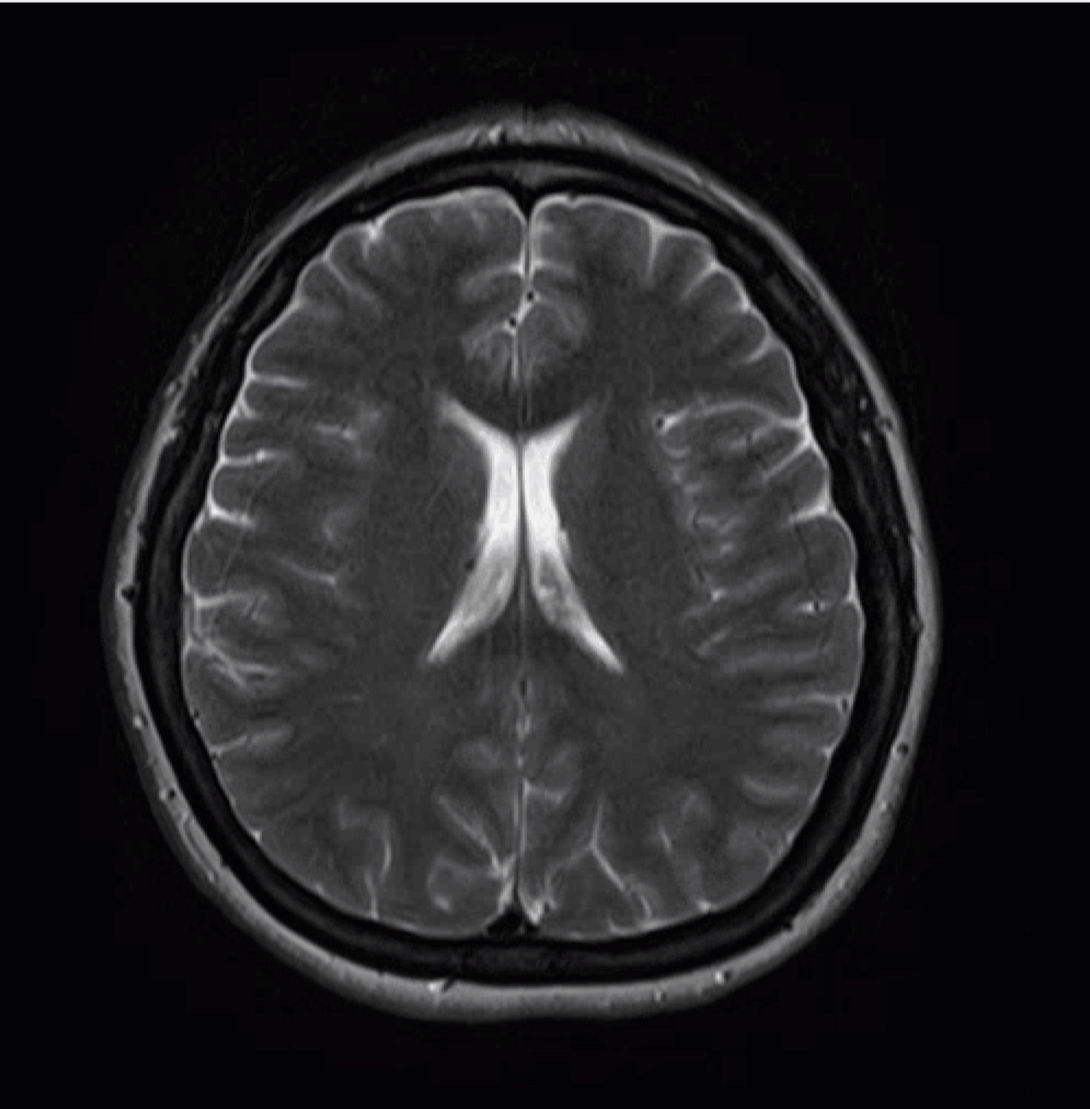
MRI brain without contrast in T2W axial view showing a normal MRI with no evidence of focal diffusion restriction to suggest acute or subacute infarction, masses, midline shift, or hemorrhages. T2W: T2-Weighted.
